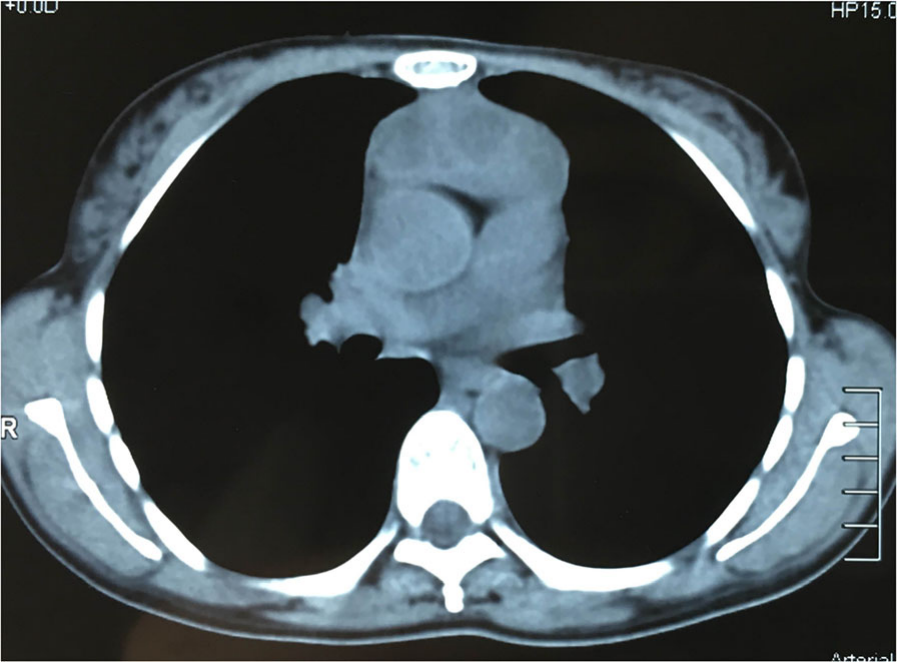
Computed tomographic findings: chest computed tomogram showed an anterior mediastinal mass composed of multilocular cyst
